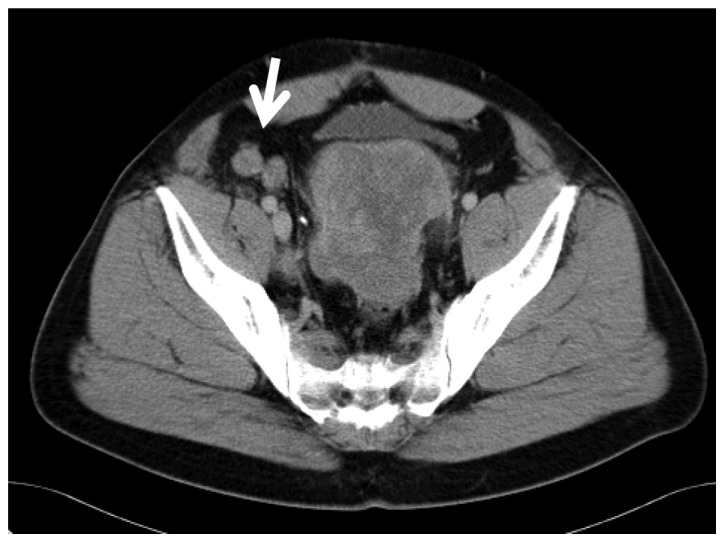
Abdominal enhanced computed tomography of the pelvis demonstrating a large mass with numerous nodules (arrow).
